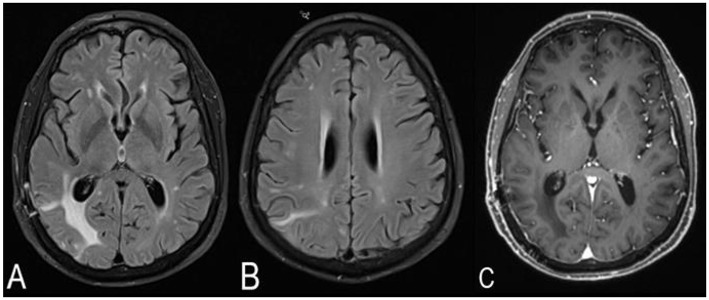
MRI brain without and with contrast 1 month after the brain biopsy and treatment with high-dose corticosteroids. The right cerebral T2-weighted fluid-attenuated inversion recovery (T2/FLAIR) hyperintensities have decreased in size, and the midline shift has resolved (A,B). No contrast enhancement is noted (C).
